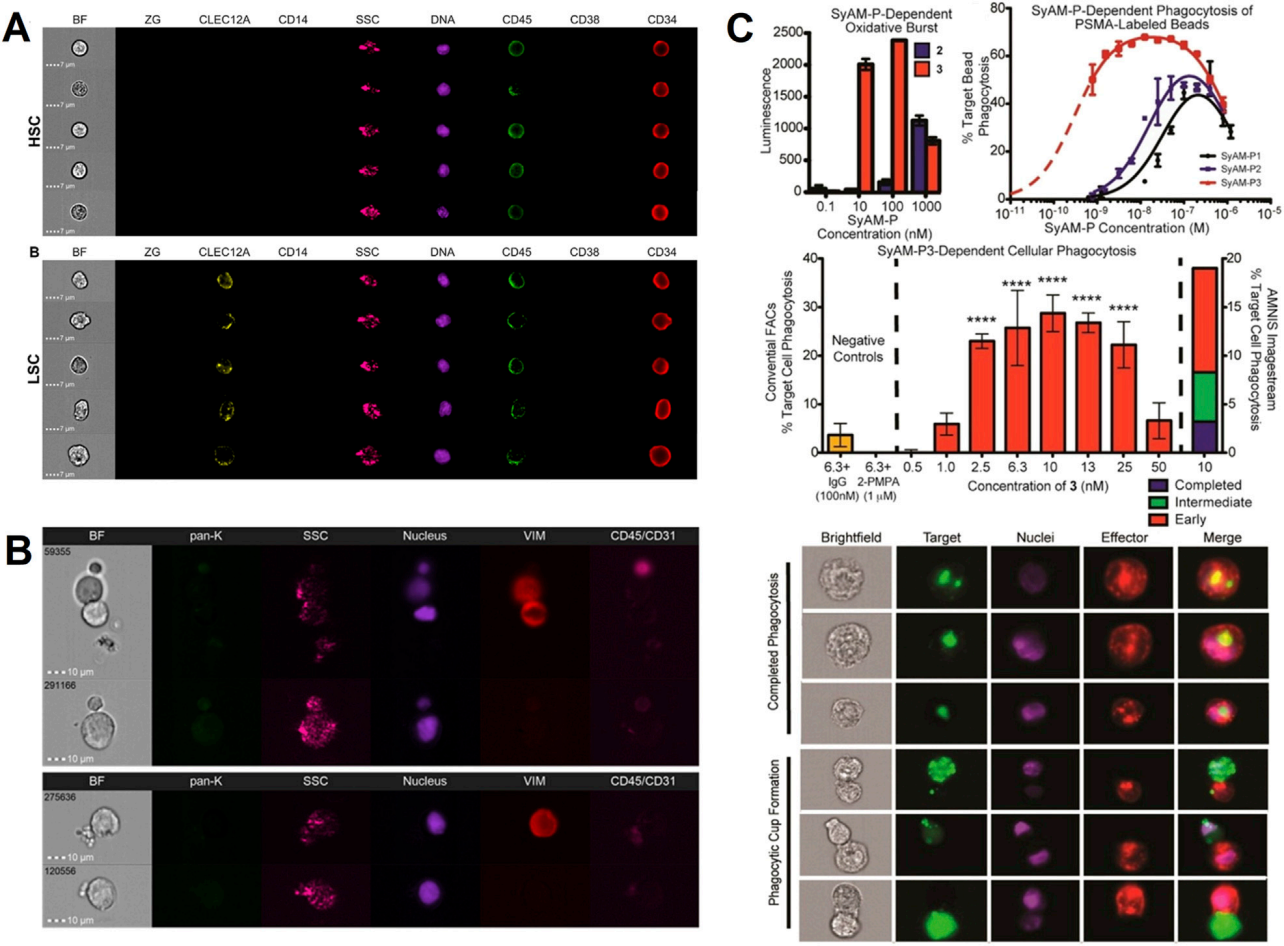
The IFC applications in oncology. (A) Leukemic stem cell identification: IFC combined with AI-based image analysis differentiates hematopoietic stem cells (HSCs) from leukemic stem cells (LSCs) in acute myeloid leukemia by morphological features (e.g., nuclear irregularity, surface marker expression) (Hybel et al., 2024). Copyright ^©^ Multidisciplinary Digital Publishing Institute. (B) Circulating tumor cell (CTC) characterization: Brightfield and fluorescent images of CTCs from prostate cancer patients highlight morphological heterogeneity. These images aid in understanding tumor metastasis mechanisms (Muchlińska et al., 2022). Copyright ^©^ Multidisciplinary Digital Publishing Institute. (C) CAR-T therapy efficacy assessment: SyAM-P molecules guide monocytes to phagocytose prostate cancer cells labeled with PSMA (prostate-specific membrane antigen). IFC visualizes and quantifies phagocytosis efficiency by tracking fluorescently labeled target cells, enabling objective evaluation of immunotherapeutic interactions (McEnaney et al., 2014). Copyright ^©^ American Chemical Society.
